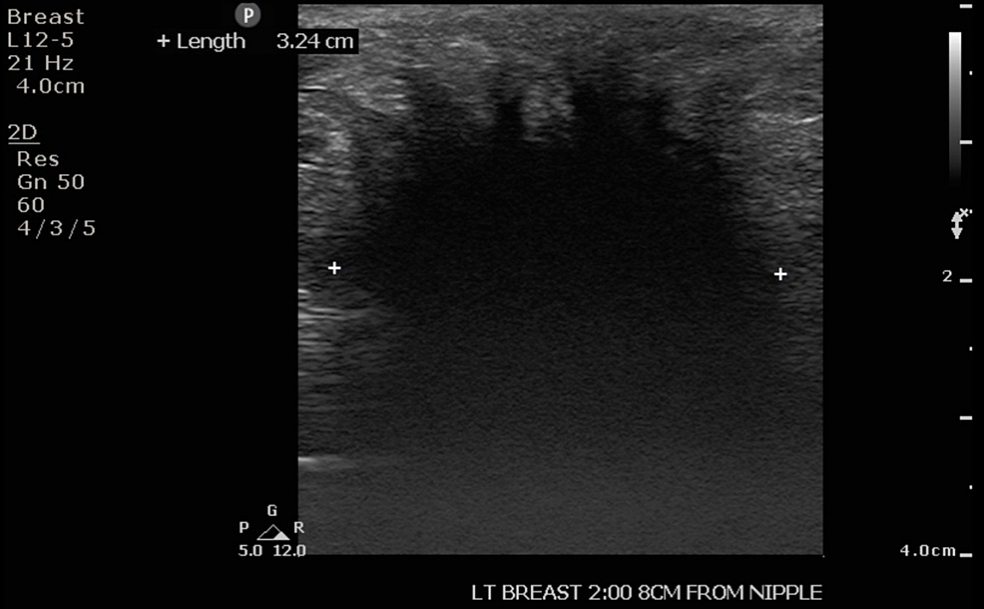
Ultrasound image of the left axillary accessory breast granular cell tumor.
